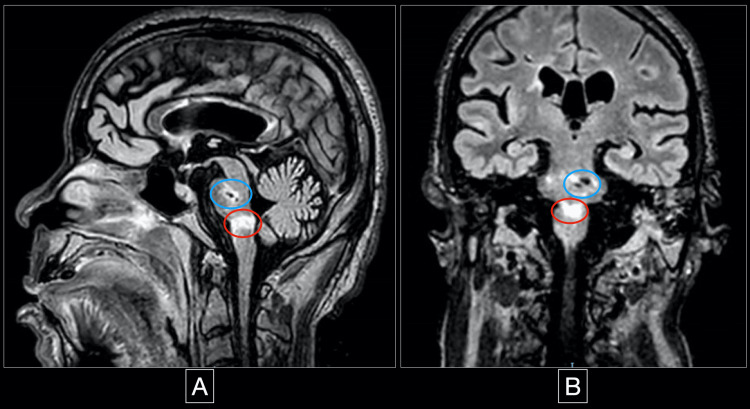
Sagittal and coronal cuts of the same brain MRI showing the acute stroke and encephalomalacia of the left pons Figure A is the sagittal view and figure B is the coronal view of the same brain MRI, this time on fluid-attenuated inversion recovery (FLAIR) sequence, and both of them show the acute medullary stroke in the red circle and a left pontine encephalomalacia consistent with an old stroke in the blue circle.
